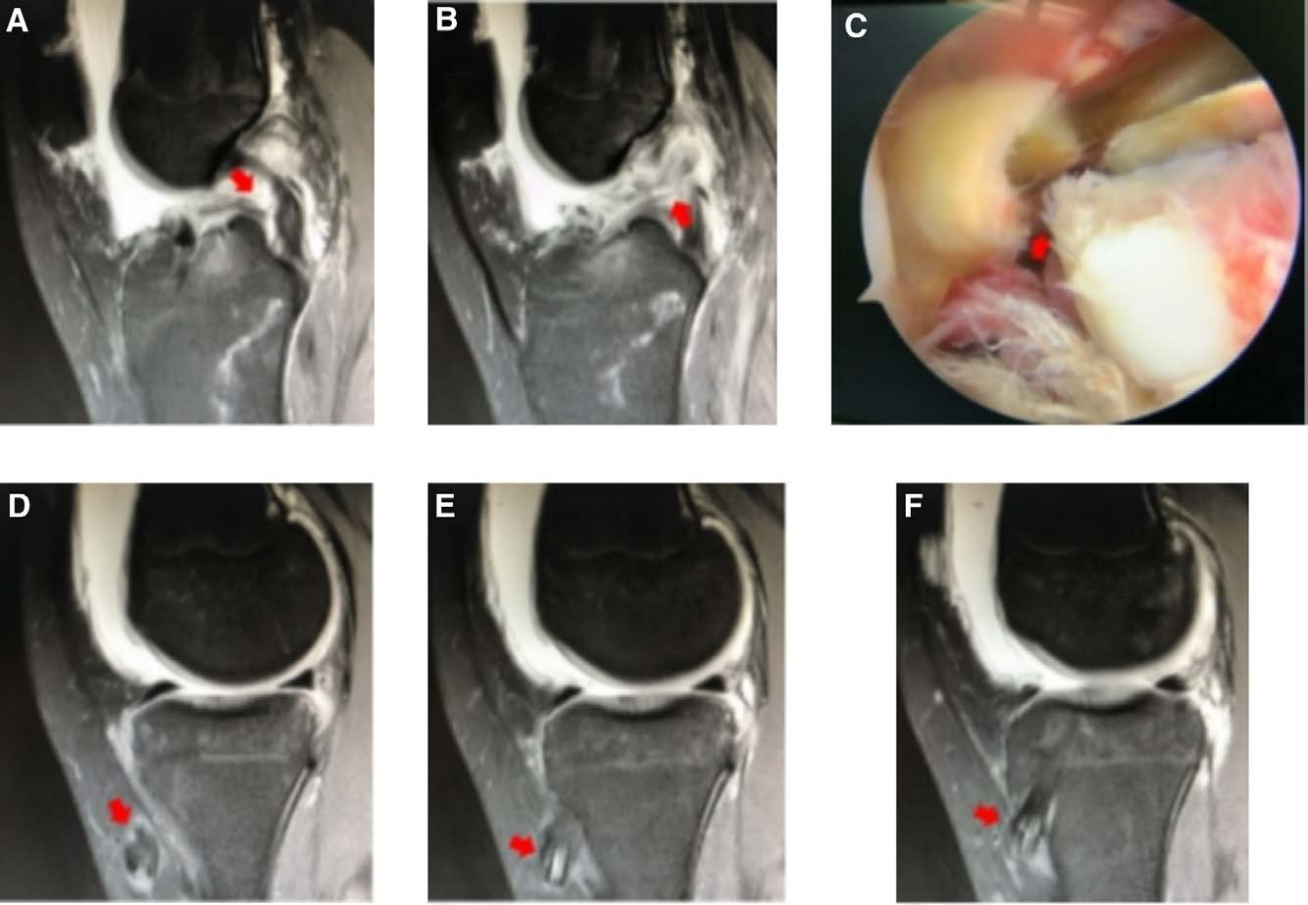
(A and B) MR: complete rupture of the ACL in the right knee. (C) Intraoperative image showing that the ACL graft had ruptured. (D–F) MRI findings showed that the tibial extrusion nail was not fully screwed into the bone canal. ACL = anterior cruciate ligament.
